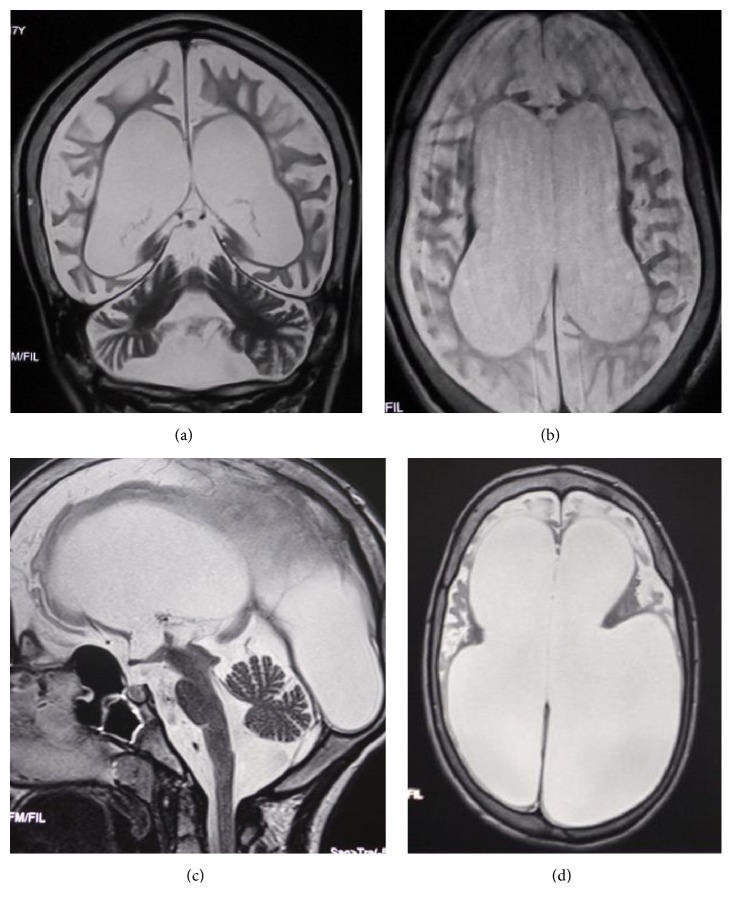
Brain MRI of the patients V.1 ((a) and (b)) and V.2 ((c) and (d)). (a) Coronal T2 weighted image showing enlargement of the cortical furrows and the ventricular cavities related to a diffuse cortical and subcortical atrophy. (b) Axial T2 weighted sequence showing the huge cortical and subcortical atrophy. (c) T2 weighted sequence shows dilatation in all ventricular cavities as a result to a diffuse and severe atrophy in the brainstem and the cerebellar and the sustentorial area. (d) T2 weighted sequence showing a major ventricular dilatation due to the severe cortical atrophy.
